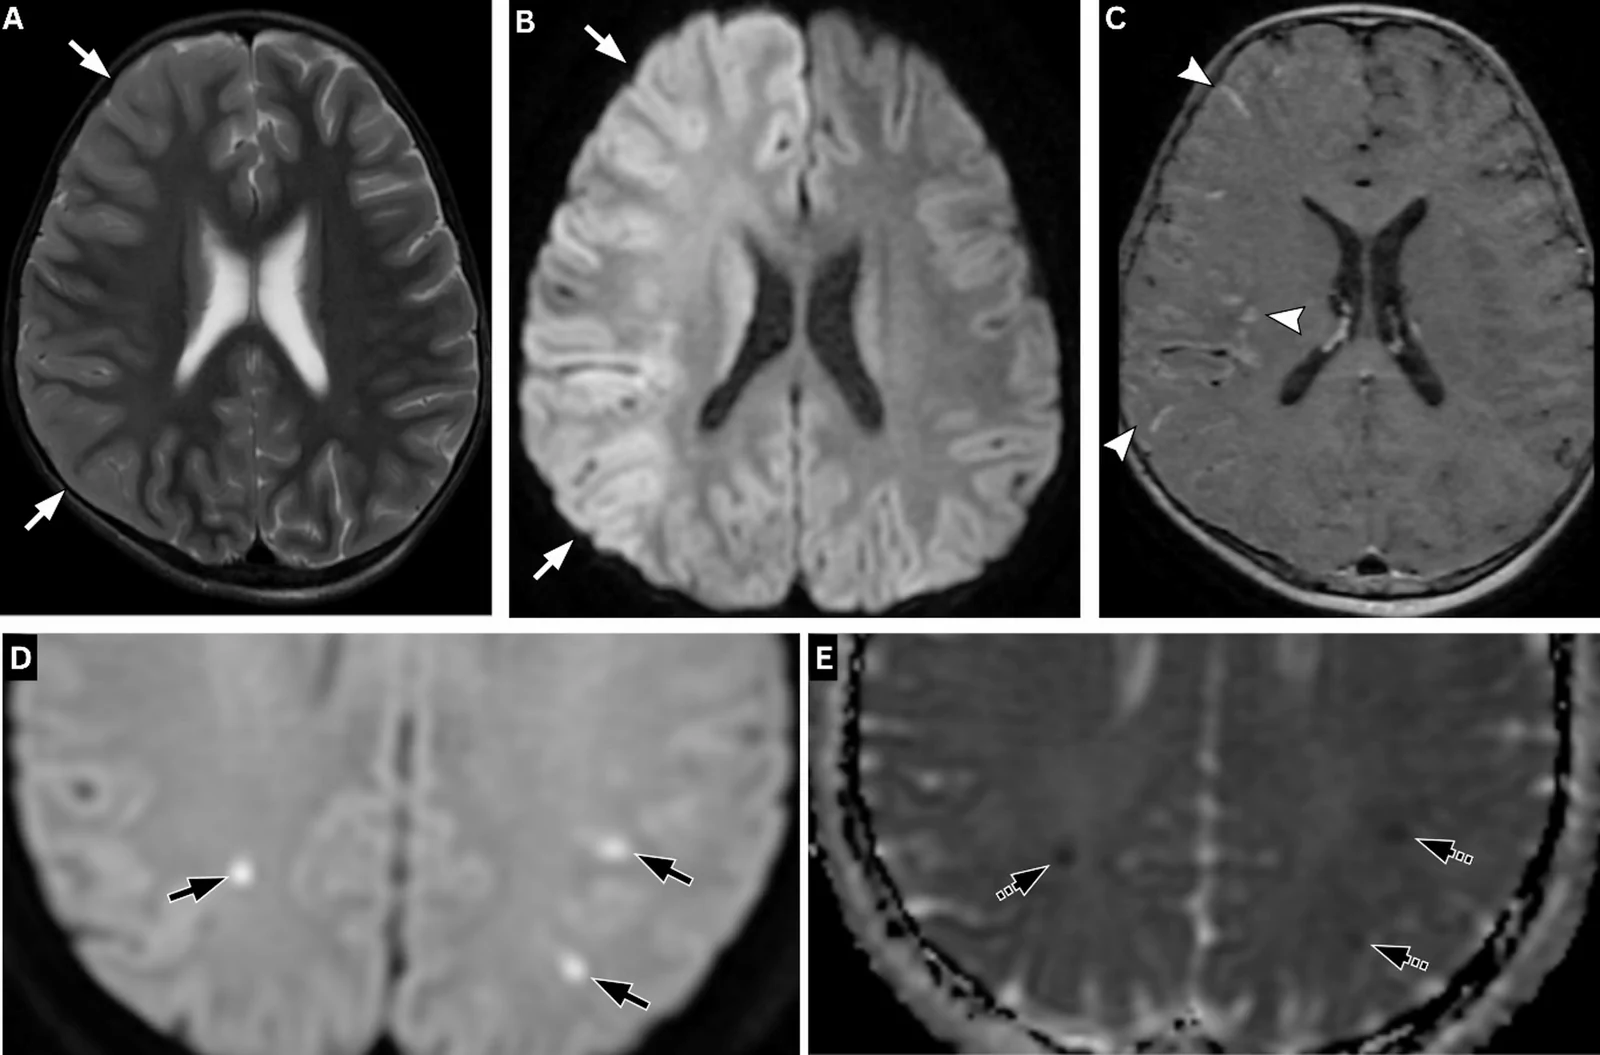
Meningoencephalitis (A–C) secondary to scrub typhus in a 5-year-old male with 5 days of fever and seizures. Axial T2-weighted image (A) and DWI (B) show gyral thickening with increased signal in the right fronto-parietal region (solid white arrows) with corresponding decreased signal on ADC map (not shown). Axial T1 fat-suppressed post-contrast (C) demonstrates leptomeningeal enhancement in the same region (white arrowheads). Vasculitis (D, E) in scrub typhus in a 49-year-old female with fever and altered sensorium for 3 days. Axial DWI (D) and ADC (E) show multiple punctate foci of diffusion hyperintensity (solid black arrows) with corresponding ADC signal drop (white arrows), consistent with vasculitic infarcts.
